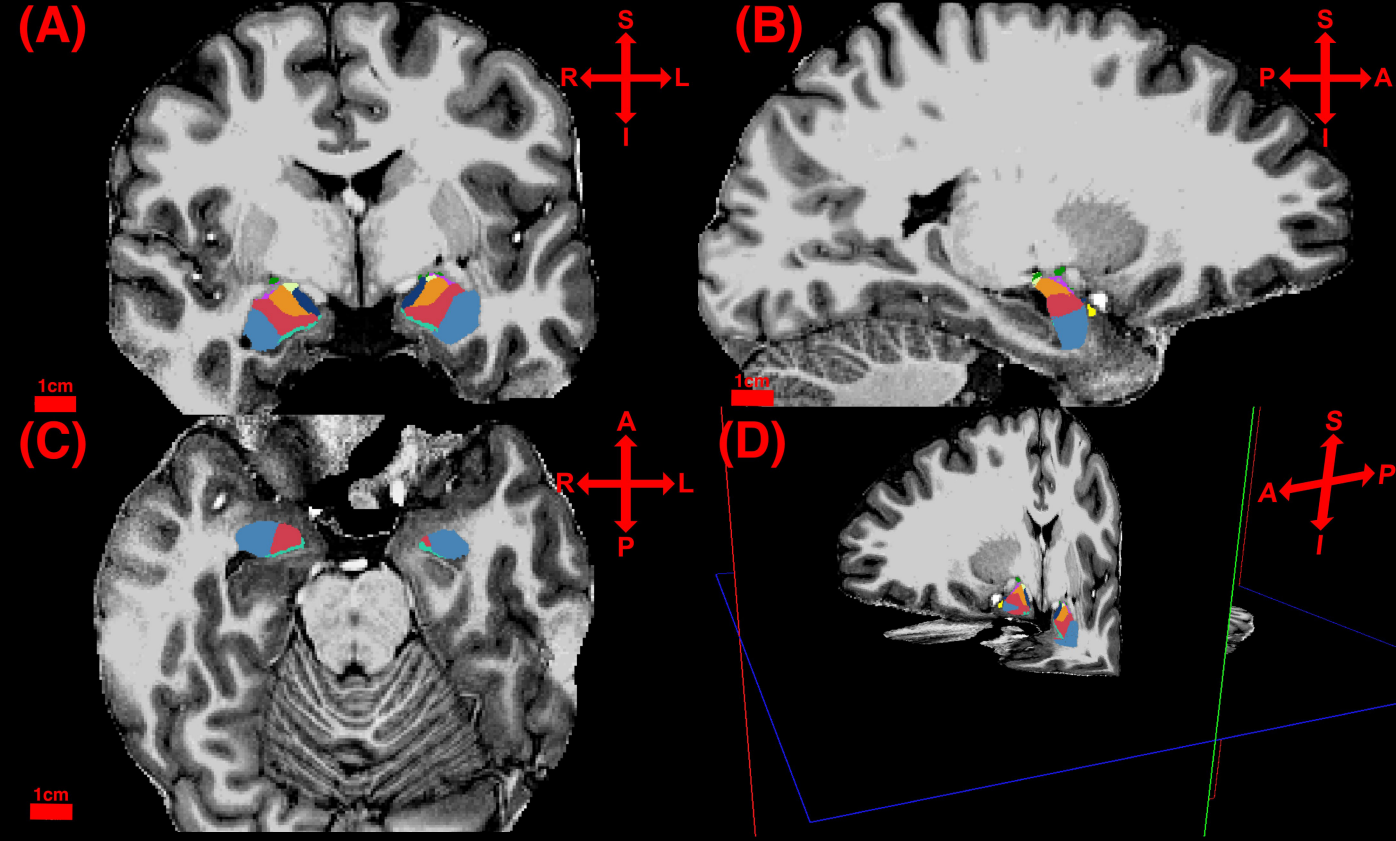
T1-weighted images at a resolution of 0.7 x 0.7 x 0.7 mm, depicting amygdala nuclei segmentation in coronal (A), sagittal (B), and axial (C) orientations, as well as in three-dimensional (3D) planar view (D). Blue indicates the lateral nucleus, red the basal nucleus, and orange the accessory basal nucleus. A, Anterior; I, Inferior; L, Left; P, Posterior; R, Right; S, Superior.
